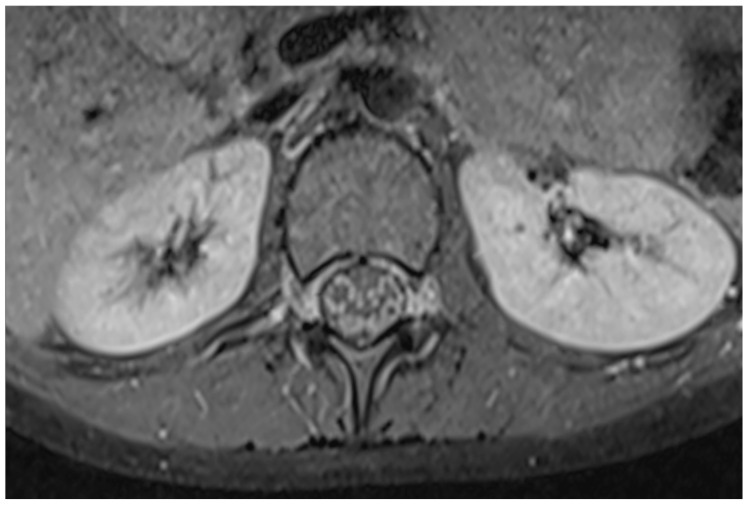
Post-contrast T1-weighted MRI of the lumbar spine demonstrating diffuse, symmetric enhancement of the anterior and posterior nerve roots of the cauda equina and lumbar spinal nerves. This imaging finding reflects breakdown of the blood-nerve barrier and increased vascular permeability secondary to inflammatory demyelinating radiculoneuropathy, as seen in Guillain–Barré syndrome. The enhancement is indicative of active inflammation and supports the diagnosis in the appropriate clinical context.
